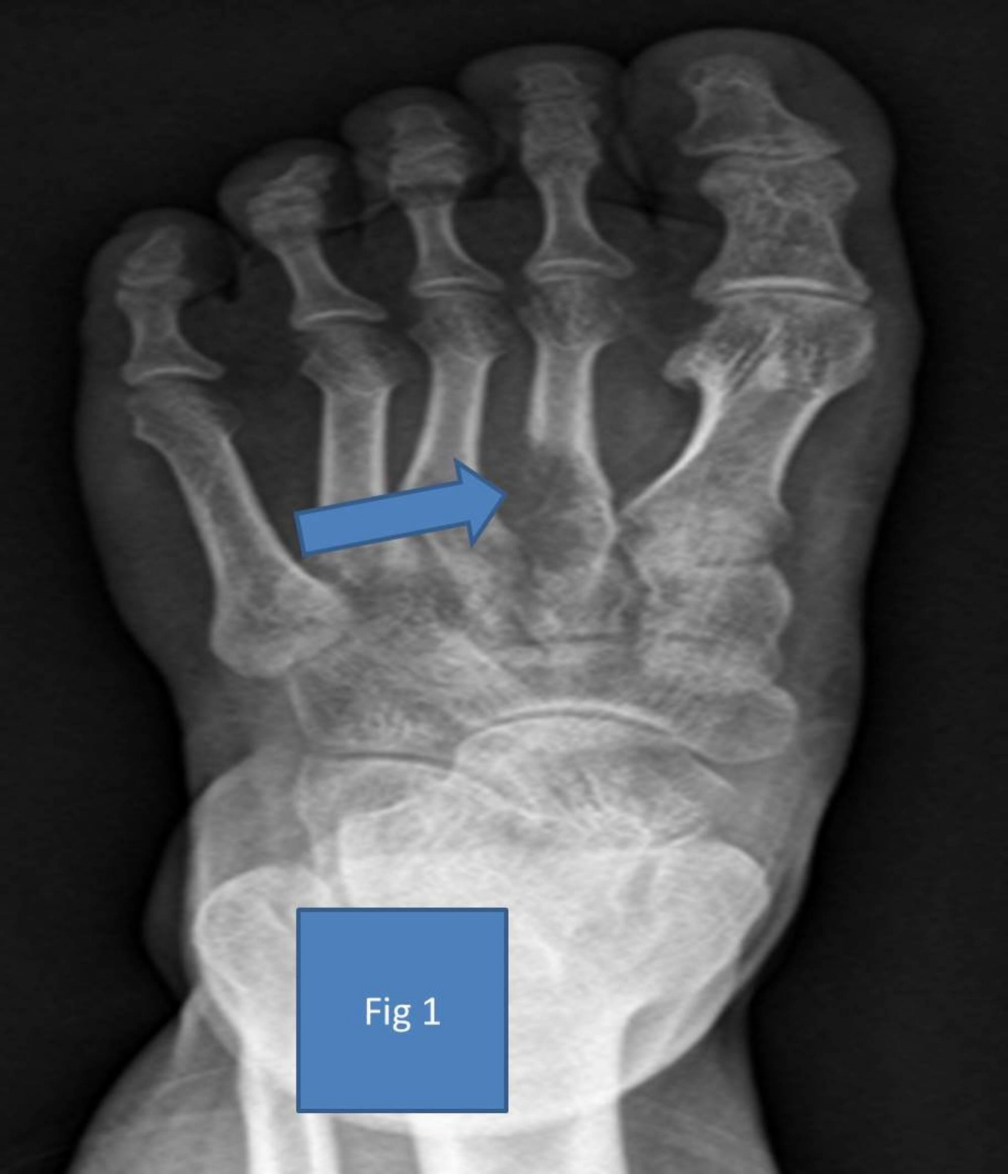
Pre-operative radiograph showing a lytic lesion in the epiphysio-metaphyseal region of the second metatarsal
